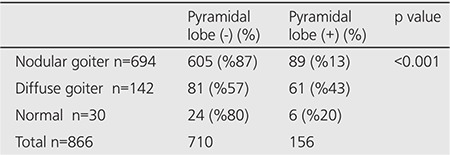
Distribution of patients according to the visualization of the pyramidal lobe n the presence of diffuse goiter, nodular goiter, and normal thyroid scintigraphy
